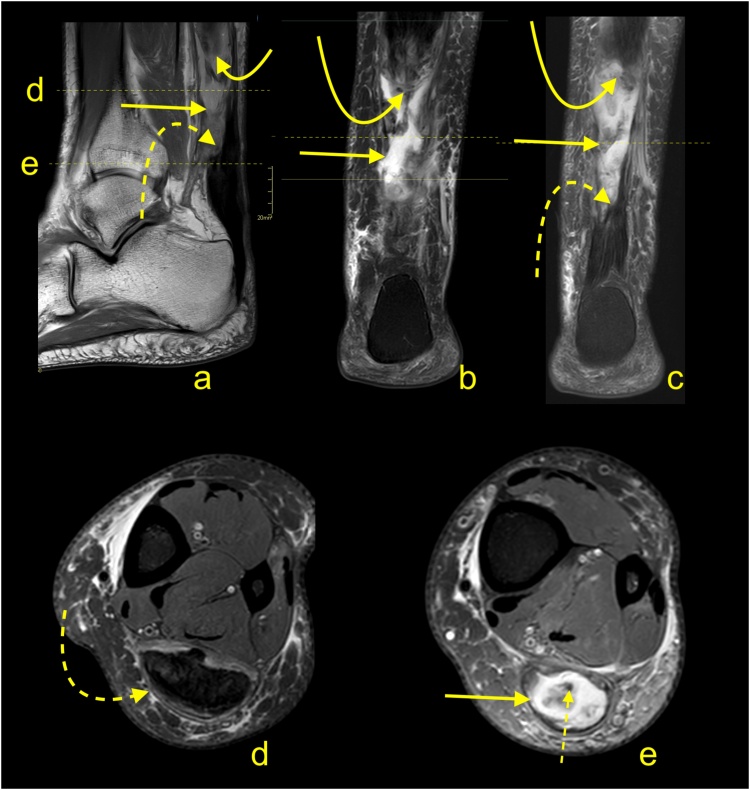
Achilles tendon rupture in midportion (straight arrow). MRI preformed 9 months after the trauma (a–e). No adhesions are visible between the proximal stump (curved arrow) and distal stump (dashed curved arrow). The paratenon at the level of rupture is filled with fluid (straight arrow). The plantaris tendon is preserved (dashed straight arrow). A- PD- weighted, b and c- T2- weighted with fat suppression, d and e- PD- weighted with fat suppression.
